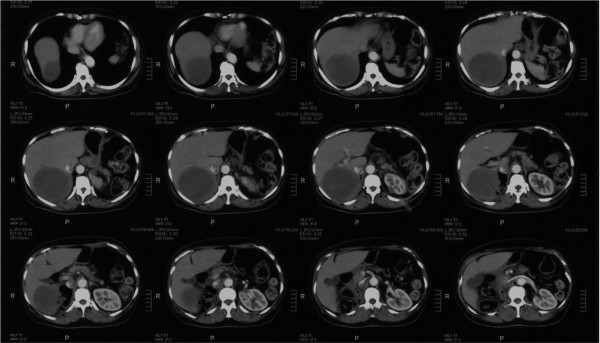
Abdominal computed tomography after sunitinib administration. The liver metastasis has liquefied and shrunk dramatically.
